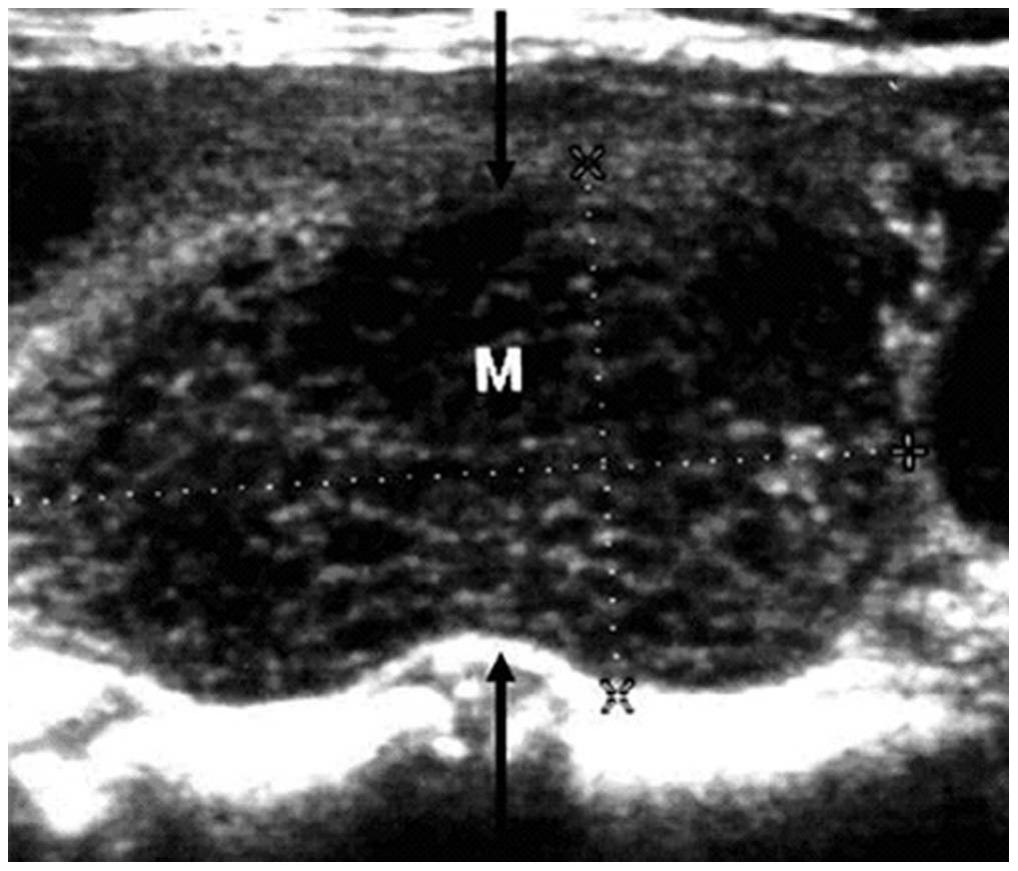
A 65-year-old female patient with non-diffuse PTL. The longitudinal sonogram shows the honeycomb appearance in a PTL lesion (arrows). (M: mass)
